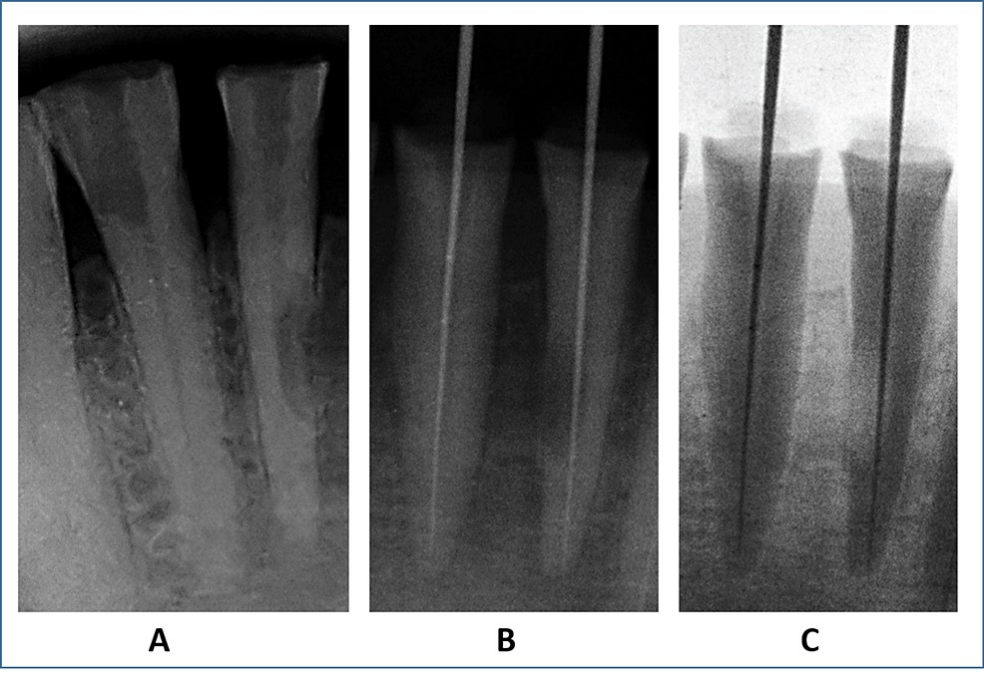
Intraoperative assessment. A: Post-gutta-percha removal radiograph. B: Working length radiograph. C: Working length radiograph inverse.
